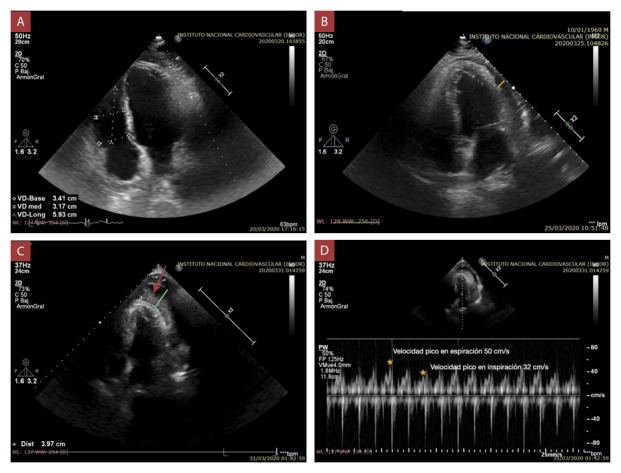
Ecocardiografía transtorácica vista apical 4 cámaras.
